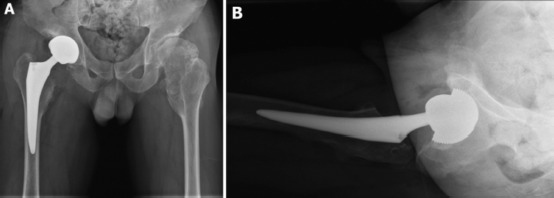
(a) Postoperative anteroposterior pelvic radiograph showing no limb length discrepancy. (b) The right lateral hip radiograph showing proper acetabular component placement.
